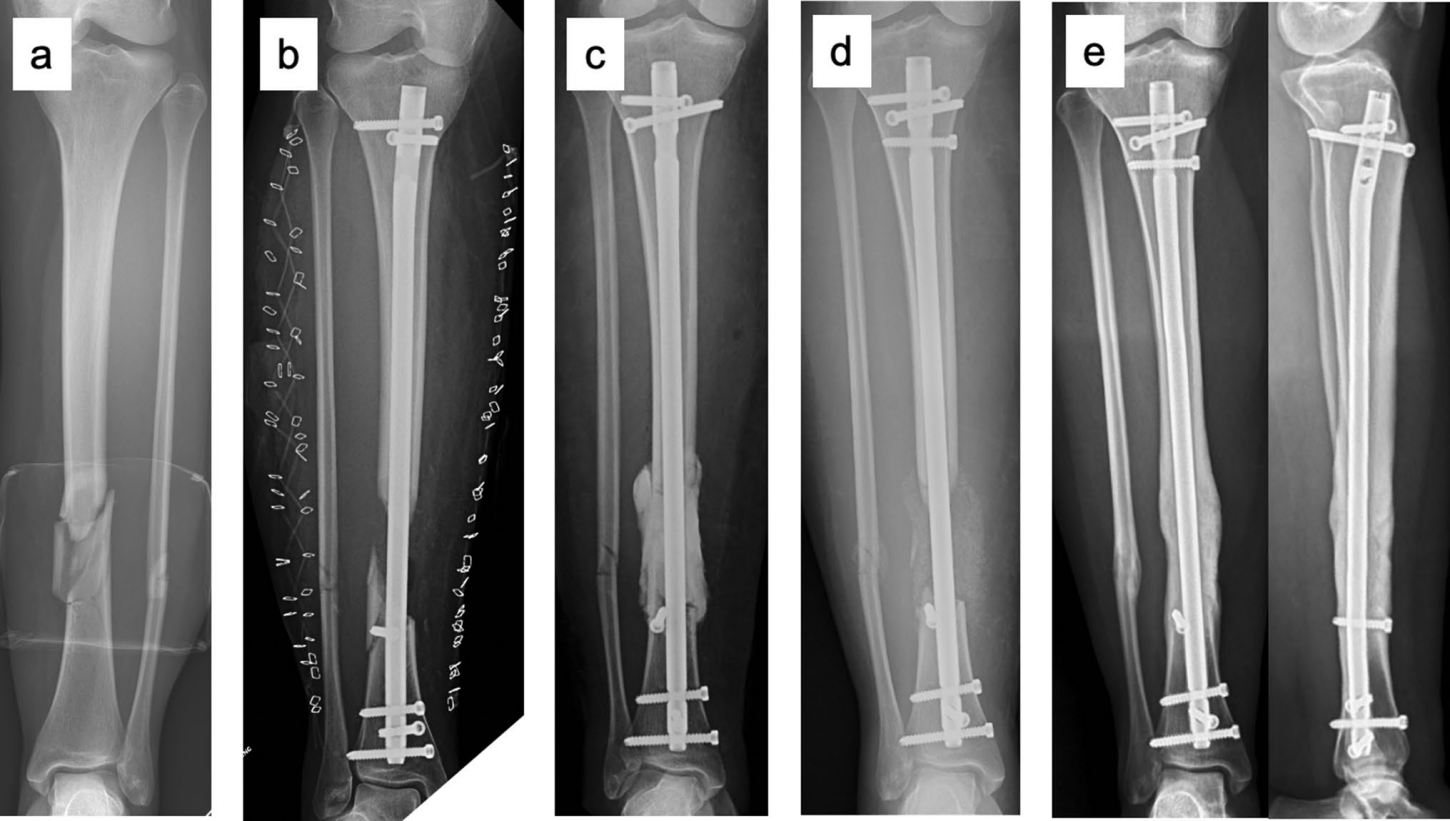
26-year-old female with a closed, segmental tibia fracture after a fall from 6 m height (a). After initial treatment with external fixation and fasciotomy, fragments without periosteal attachment were removed, and the fracture was stabilized with an intramedullary nail (b). Subsequently, the 6-cm bone void was filled with PMMA cement (c). After 38 days, the cement spacer was removed, and the void was filled with autologous bone graft (d). At follow-up 26 months after IMT stage 2, the patient had obtained good functional results and the radiographs showed complete union (e)
